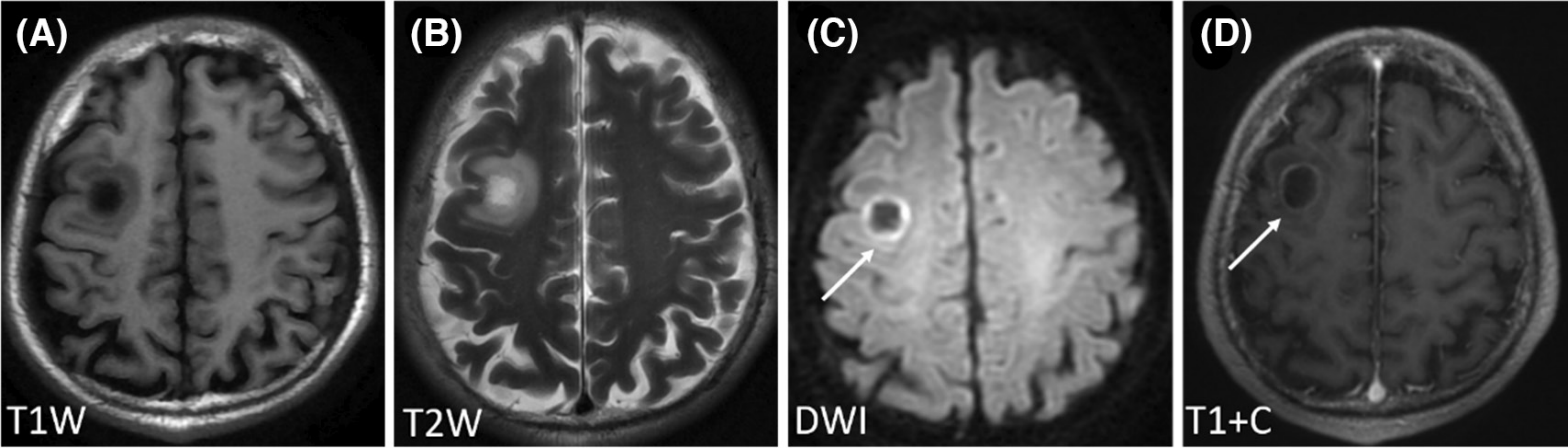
Pattern V of brain metastases in SCLC. Pattern V: A 62‐year‐old man with a history of smoking was diagnosed with BMs of SCLC. The patient had nine lesions. One lesion (white arrow) was located in the right frontal lobe, with hypointensity in T1WI (A), hyperintensity in T2WI (B), ring hyperintensity in DWI (C) and ring enhancement (D). BMs, brain metastases; DWI, diffusion‐weighted imaging; SCLC, small cell lung cancer.
